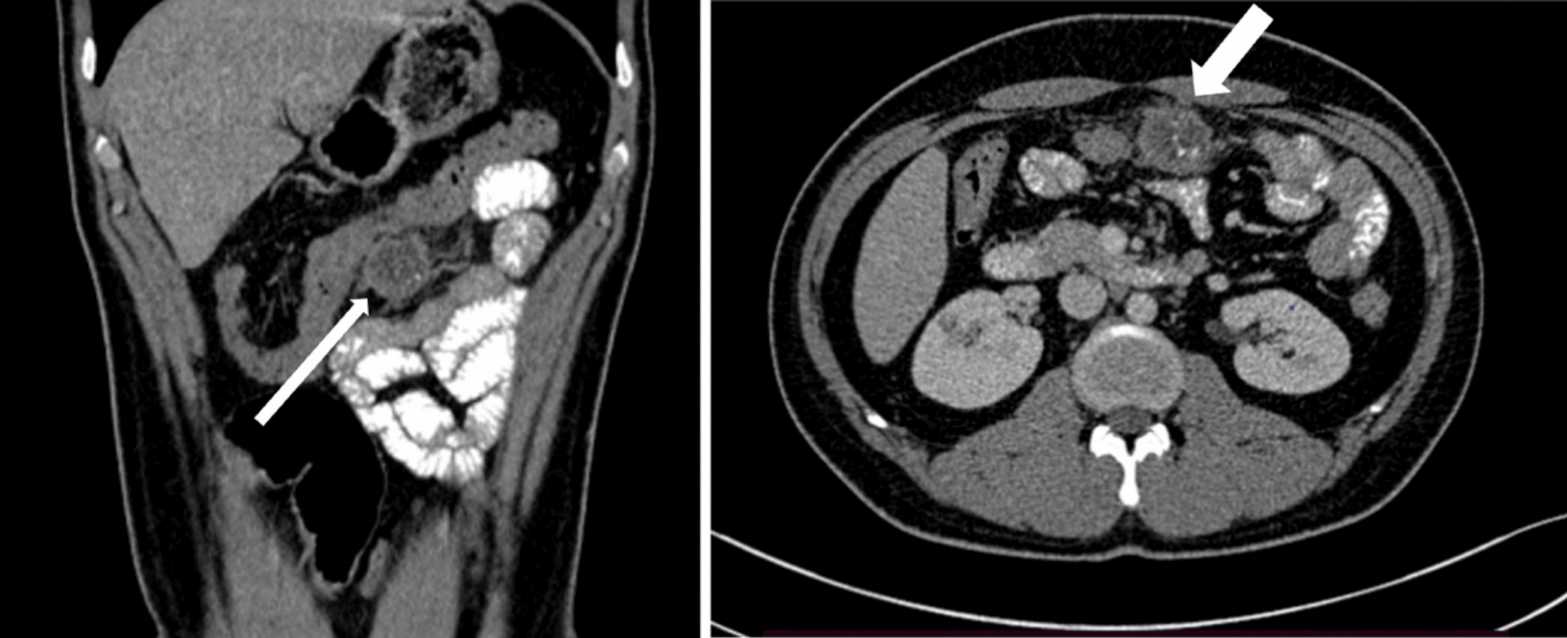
Computed tomography of the abdomen showing foci of calcification adjacent to the transverse colon, suggestive of a complex omental cyst or omental infarct CT, computed tomography
